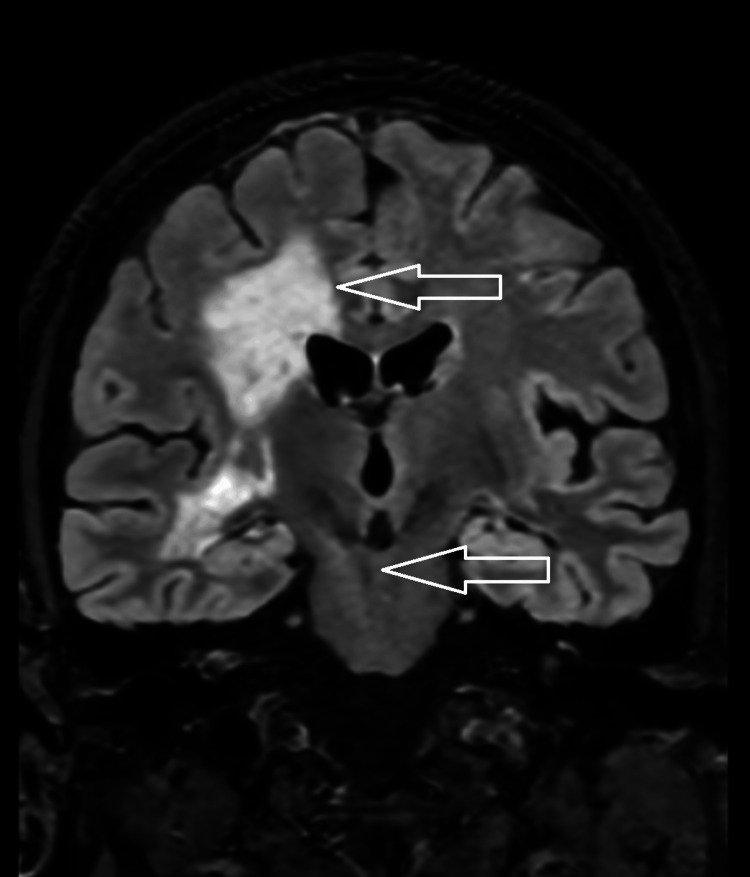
FLAIR signal with descending spread Coronal T2 fluid-attenuated inversion recovery (FLAIR) showing involvement largely within the right cerebral white matter as depicted by the superior unfilled arrow. A newly conspicuous FLAIR hyperintensity is seen within the right midbrain and pons, pointed out by the inferior unfilled arrow.
